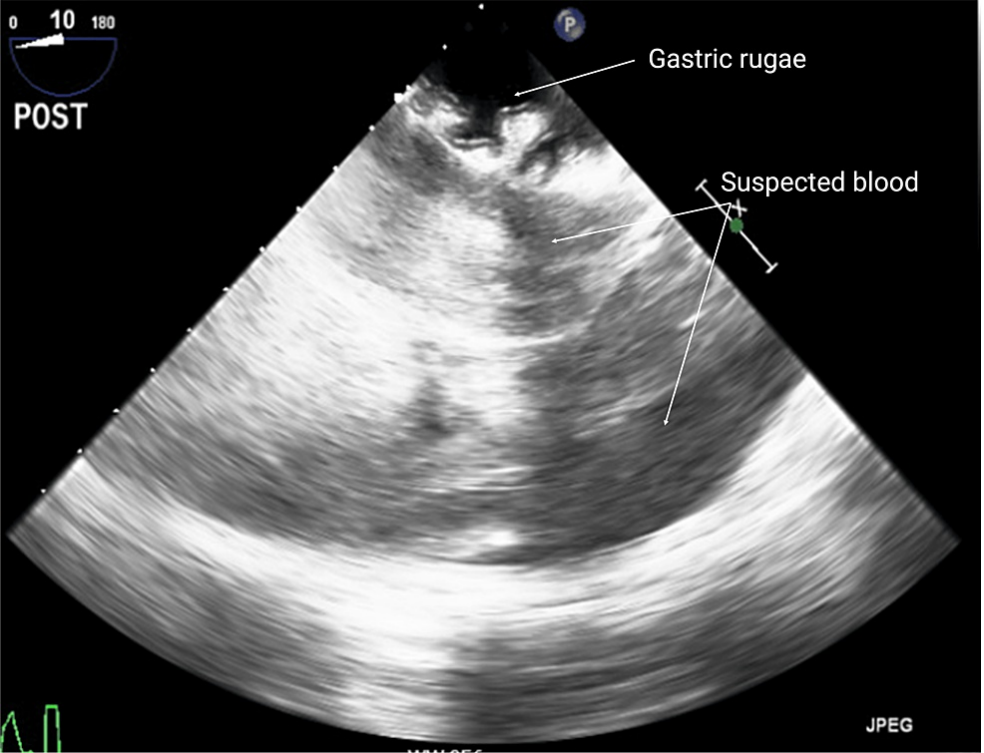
TEE view of the stomach revealing nonspecific hypoechoic areas anterior to the stomach concerning for blood accumulation TEE: transesophageal echocardiography
